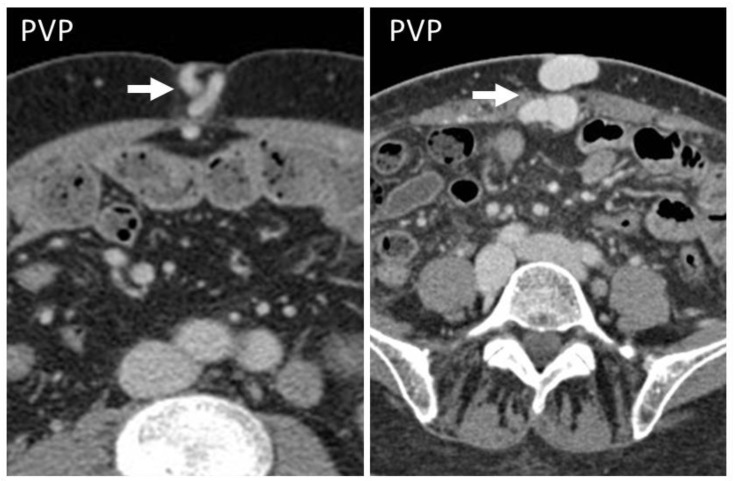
CT appearance of “caput medusae” on axial, contrast-enhanced CT scan on portal venous phase in two cirrhotic patients, as a result of the recanalization of the umbilical vein due to portal hypertension. This appearance is due to tortuous vessels (arrows) reaching the abdominal wall at the level of the navel.
